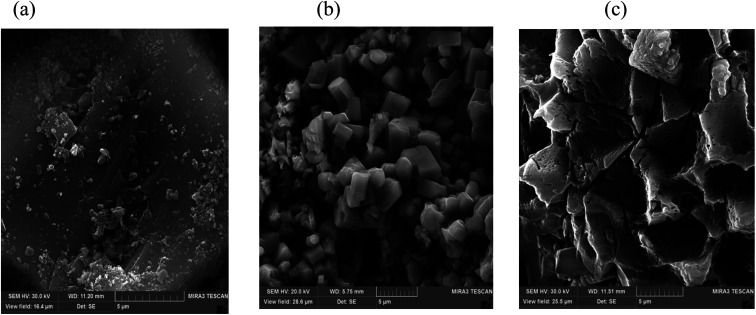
FESEM images of (a) raw ash, (b) leaching without ultrasound, and (c) leaching with ultrasound.
